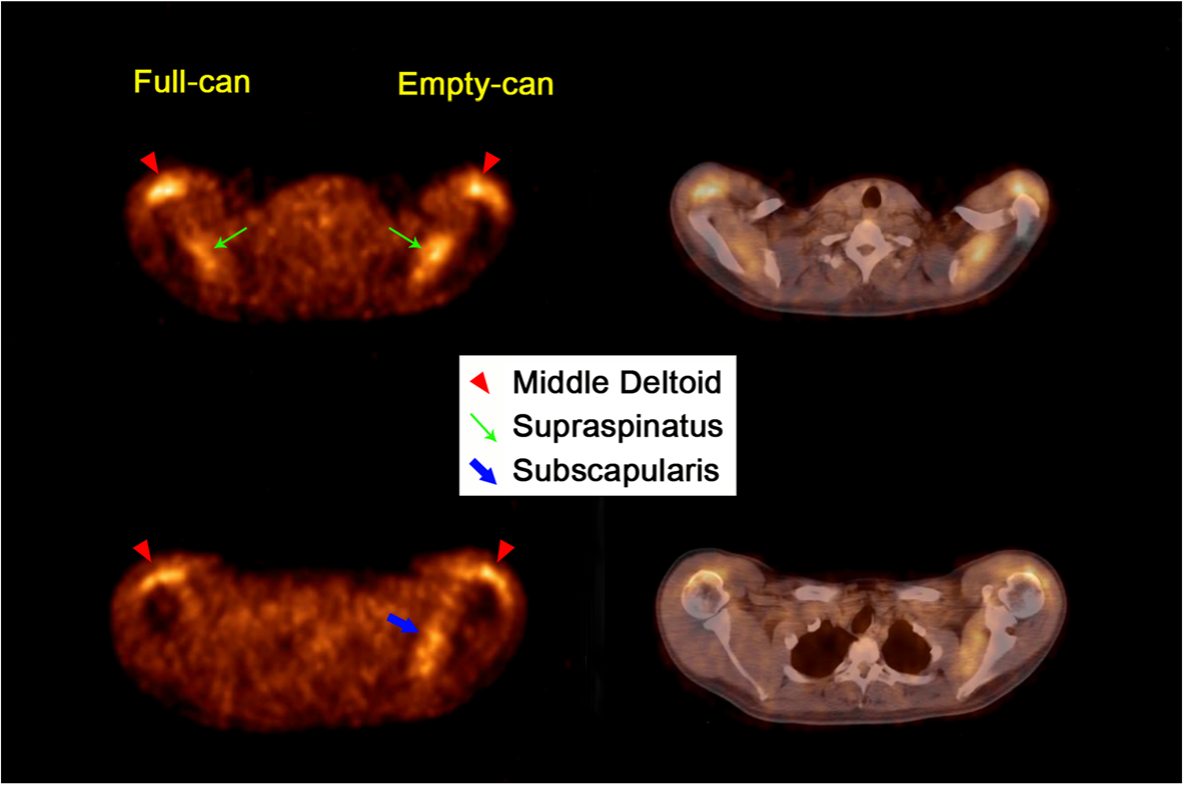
Axial views of PET/CT. The PET/CT images of one case demonstrated increased muscle activity of the middle deltoid, supraspinatus, and subscapularis after maintaining the arm in the empty-can versus the full-can testing position.
